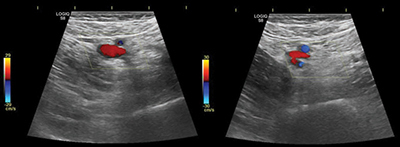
Doppler ultrasound of the left lower limb without signs of DVT.
